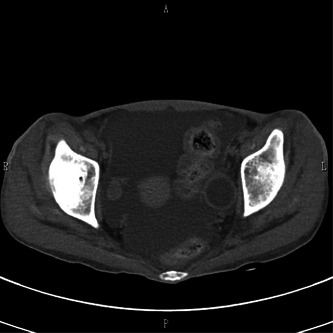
Abdominal computed tomography (transverse view). A large amount of ascites was apparent around the intestine.
